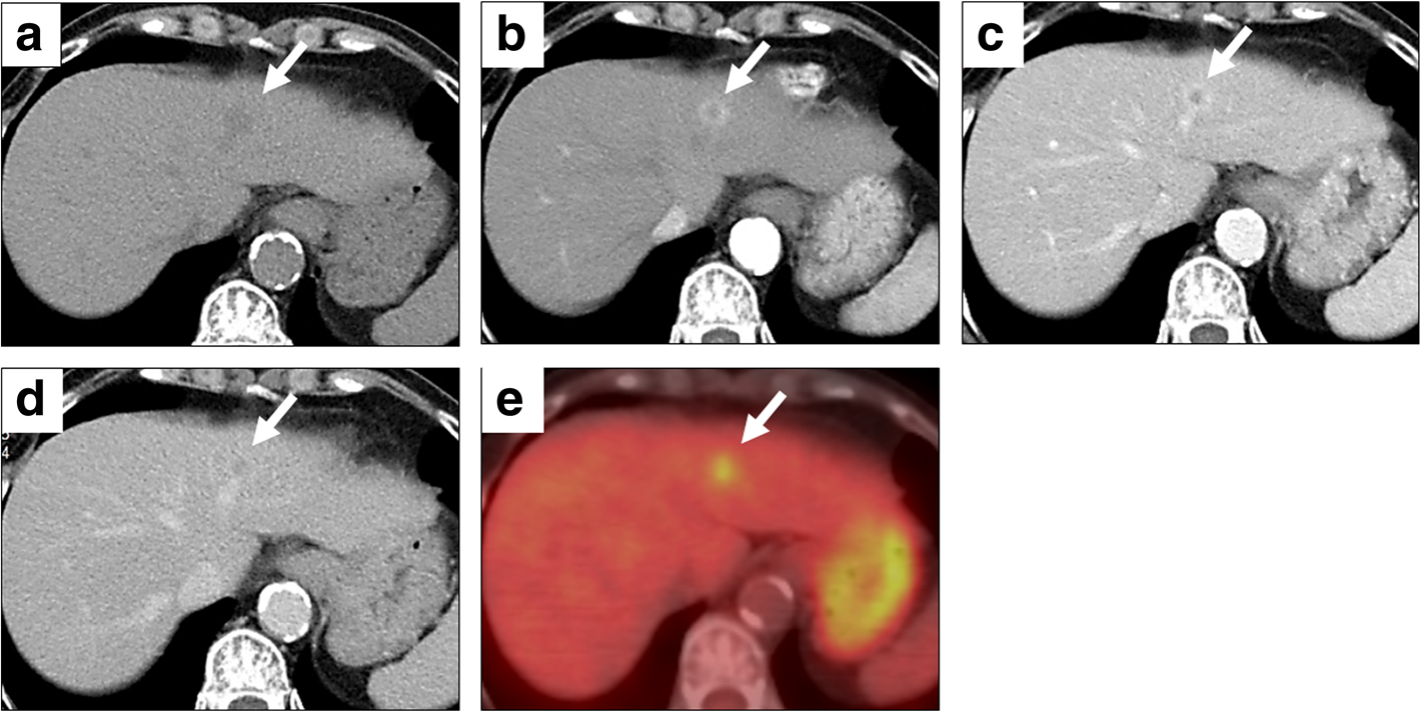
Findings of dynamic computed tomography (CT) and FDG-PET. a Conventional CT shows a low-density tumor whose margin is unclear in the left lobe of the liver. b The tumor shows marked enhancement at the periphery in the arterial phase. c The tumor shows prolonged enhancement at the periphery in the portal phase. d In late phase, the tumor shows slightly lower density relative to the normal liver but has a faint enhancement inside the tumor. e The tumor exhibits uptake of FDG, whose SUV max is 4.7
